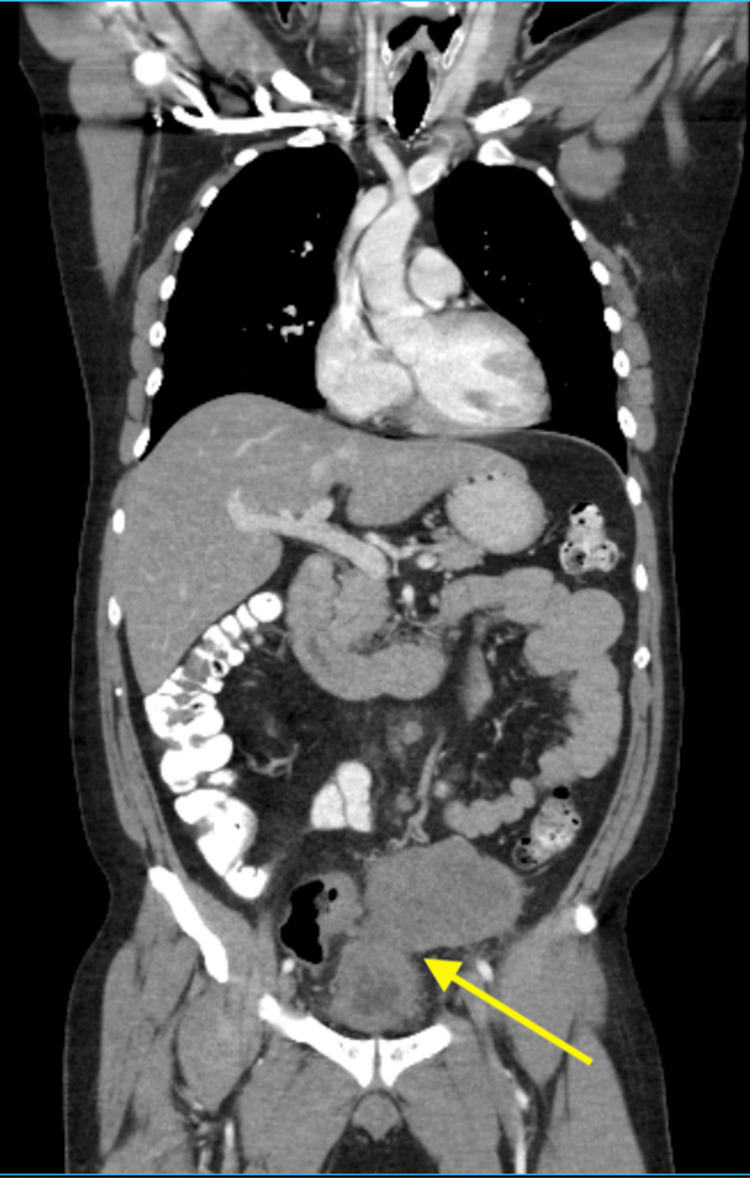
CT scan of chest, abdomen, and pelvic showing invasion of the rhabdomyosarcoma into the urinary bladder (yellow arrow)
